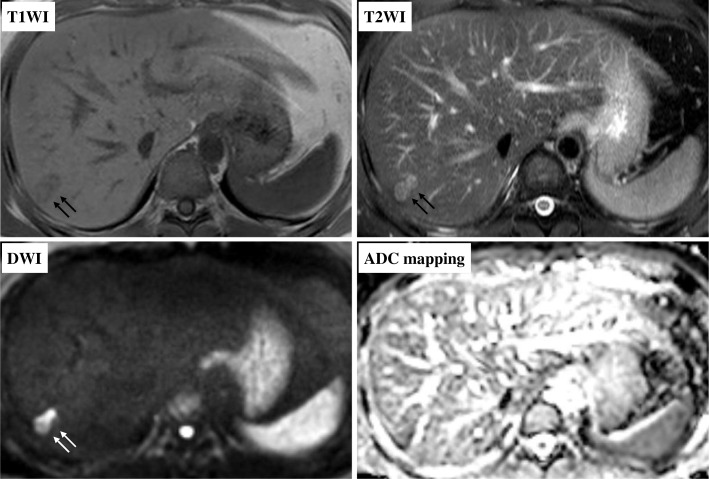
Axial slice on MRI before operation. The liver mass (dumbbell-shaped) had low signal intensity on T1-weighted images (T1WI), high signal intensity on T2-weighted images (T2WI), marked signal hyperintensity in diffusion-weighted imaging (DWI), and no signal hypointensity on ADC map
